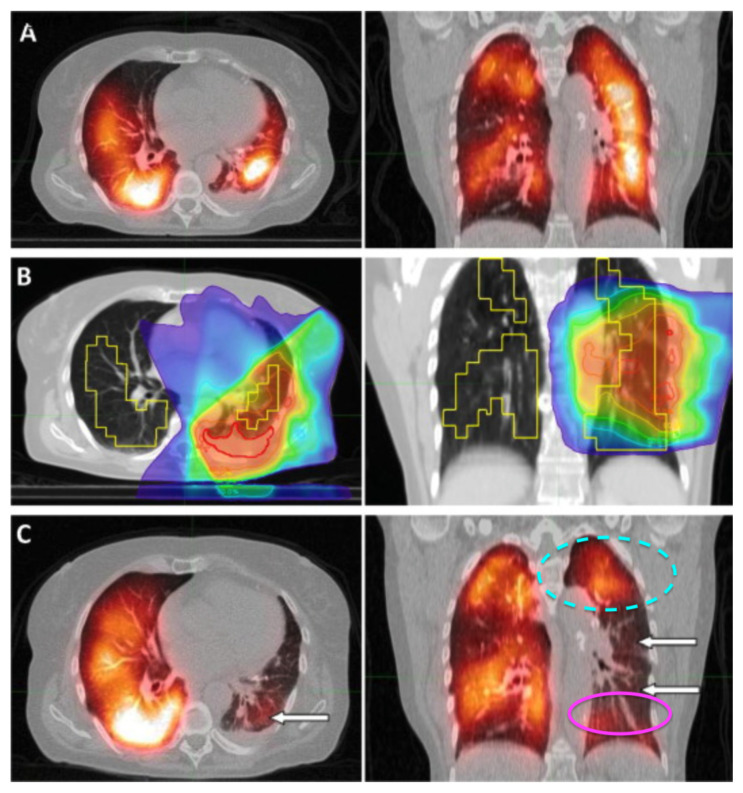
Taken from Farr et al. [25]. SPECT/CT of a patient with tumor in the right lung before radiotherapy (A), planning CT with dose to the gross tumor volume in color wash, SPECT defined functional lung outlined in yellow (B), SPECT/CT 3-months post-RT (C). A dotted cyan oval is drawn to indicate a region that received low dose but did not experience perfusion decline. A magenta oval is drawn in another region that received low dose but did experience perfusion decline post-RT and is fed by an irradiated region. Reprinted with permission.
